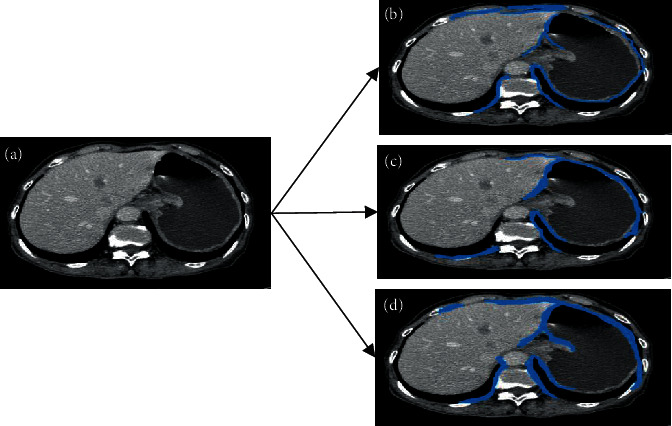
The detection results of three algorithms for suspected lymph nodes in gastric CT images. Blue indicated suspected lymph nodes: (a) original image; (b) RO-OTUS algorithm; (c) LR algorithm; (d) RO algorithm.
